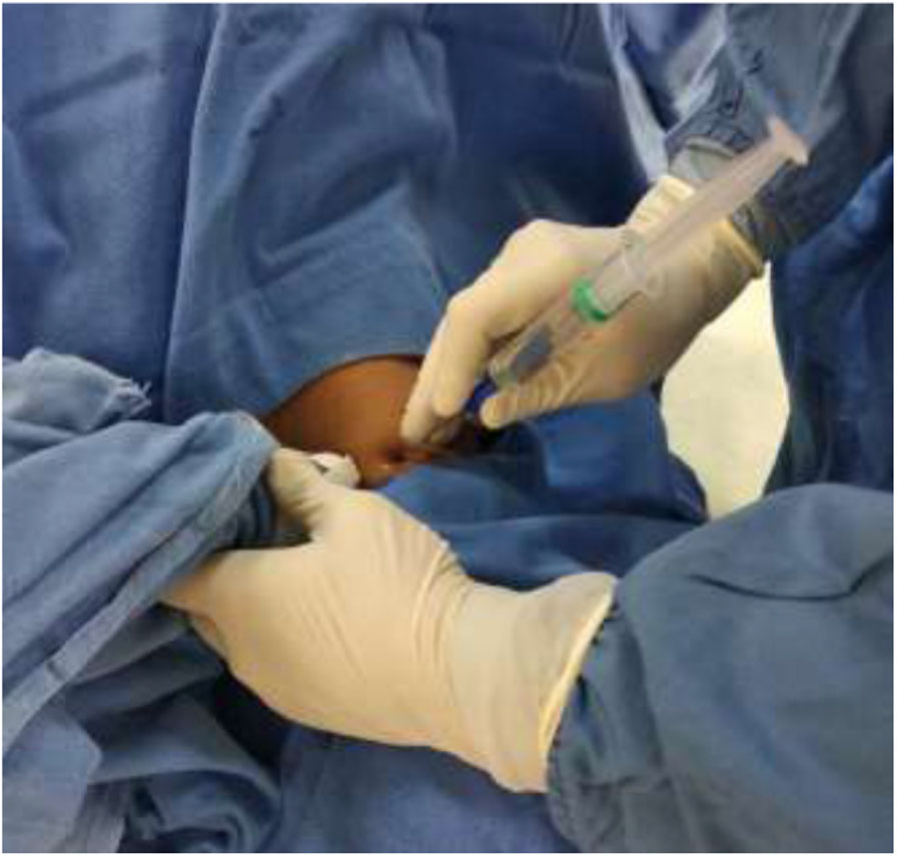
USG (Ultrasound-guided) ACB (Adductor canal block)
